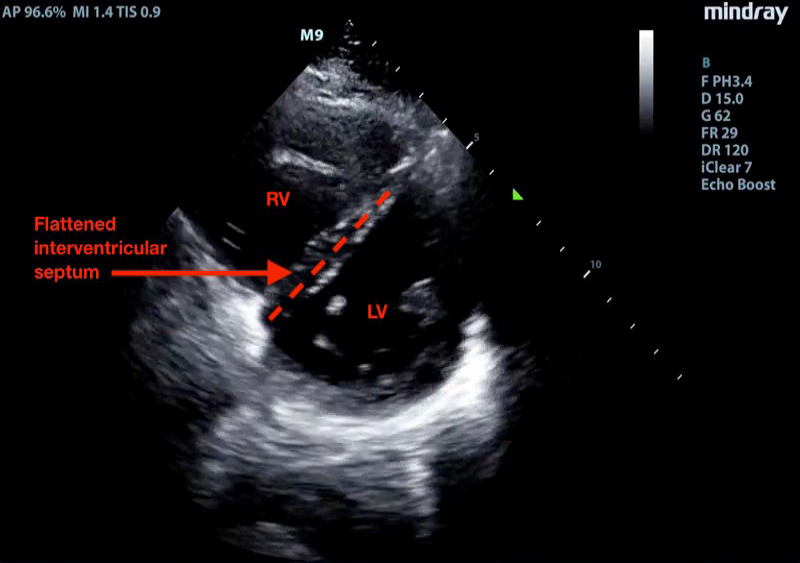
Parasternal short view of the heart, demonstrating bowing of the interventricular septum away from the right ventricle (RV) into the left ventricle (LV) (the “D-sign”).
